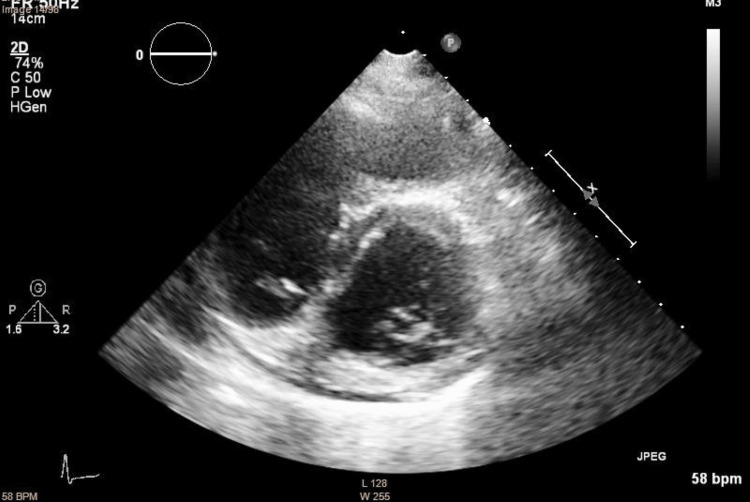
Larger right ventricle and D-sign, seen on parasternal short axis
